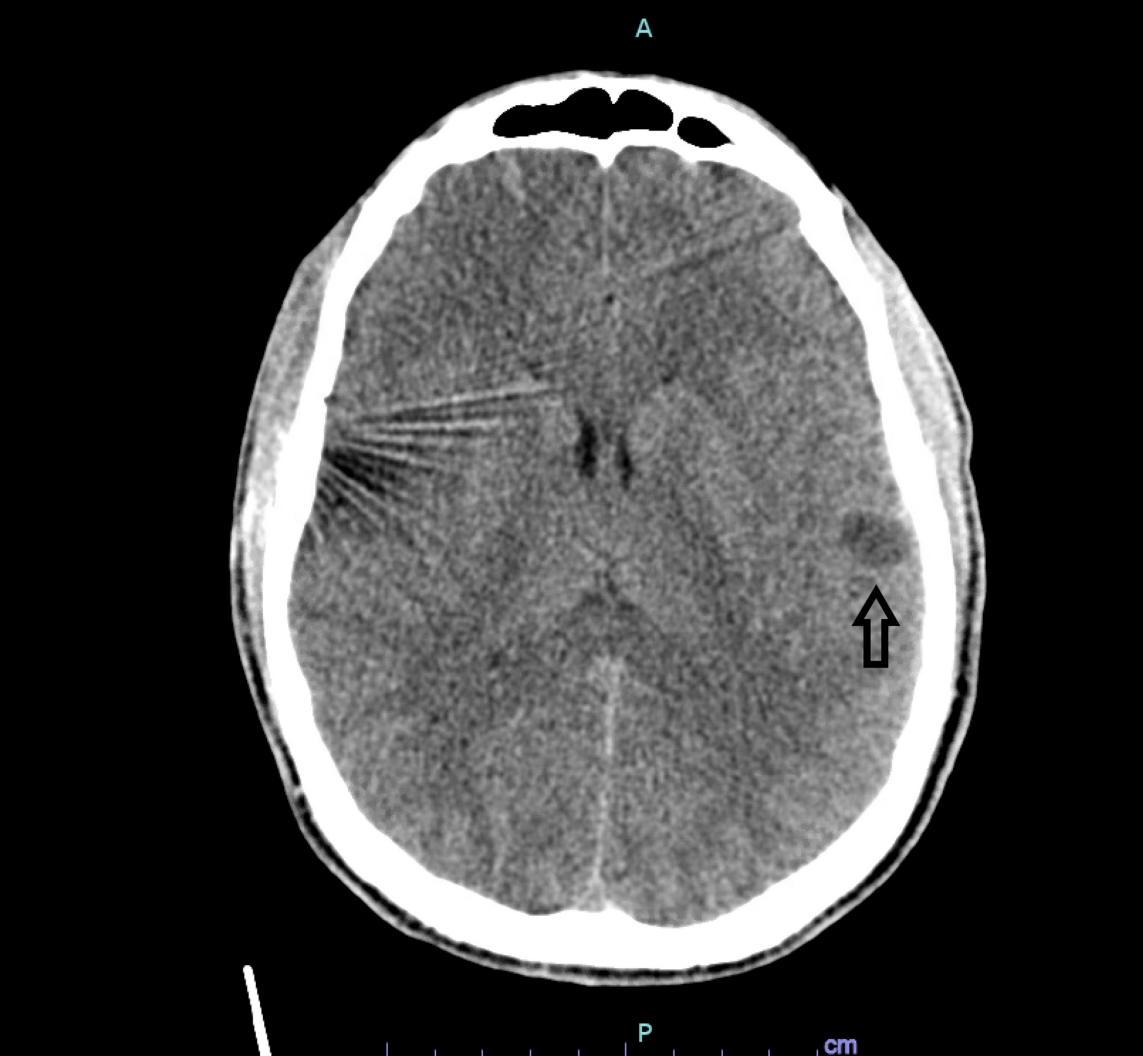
CT head demonstrating increased hypodensity in the left frontal lobe
